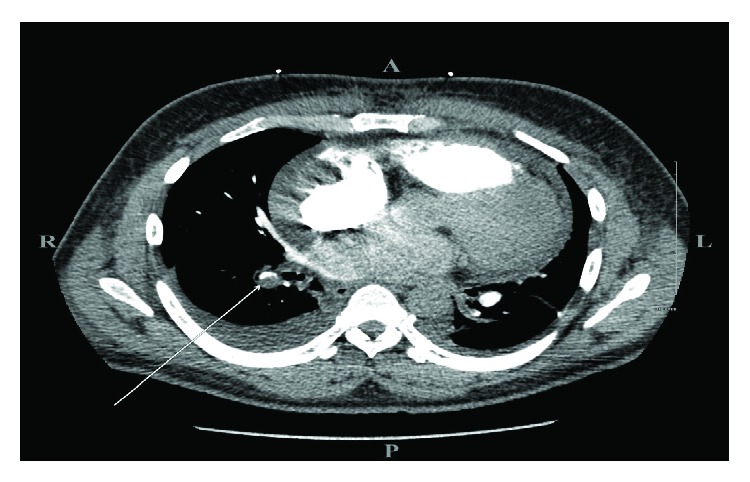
A contrast-enhanced chest computed tomography with pulmonary embolism protocol: large filling defect within the distal right lobar pulmonary artery, with thrombus extending into the right lower lobe branches (white arrow). The heart is markedly enlarged with a moderate pericardial effusion. Bibasilar consolidations and areas of linear atelectasis versus scarring/fibrosis at both lower lobes. Bilateral pleural effusions, larger on the right than the left. There is no pneumothorax or pneumomediastinum.
